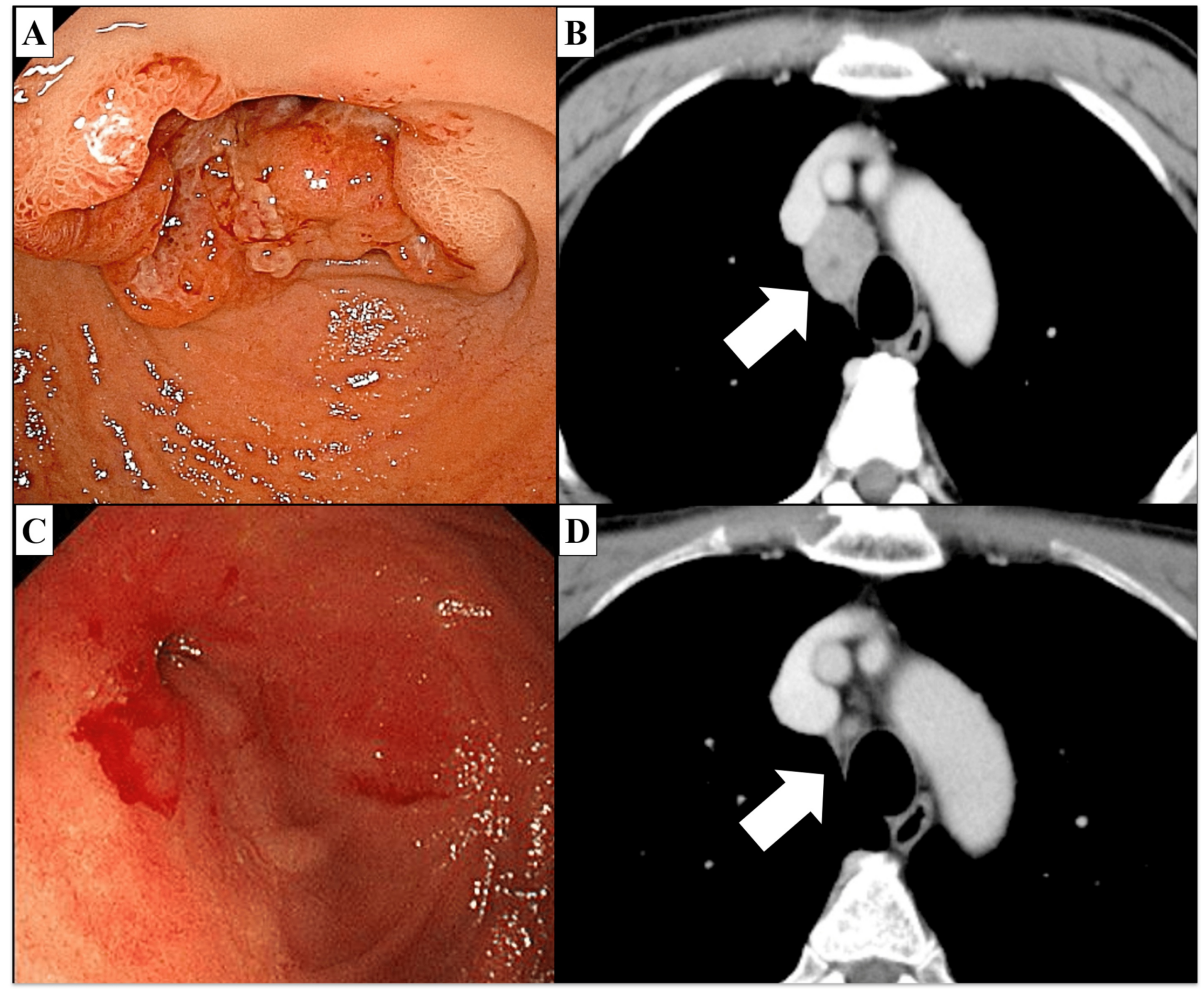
Gastroduodenoscopy and CT taken prior to and following chemotherapy. (A) Gastroduodenoscopy revealed a type 2 circumferential tumor in the descending duodenum. (B) CT imaging showed that the mediastinal lymph node had enlarged to a diameter of 3 cm. (C) Following chemotherapy, gastroduodenoscopy revealed the regression of the duodenal tumor. It was unclear whether the cancer had been entirely eradicated because a cicatricial stricture remained. (D) CT showed a reduction in the size of the mediastinal lymph node.
